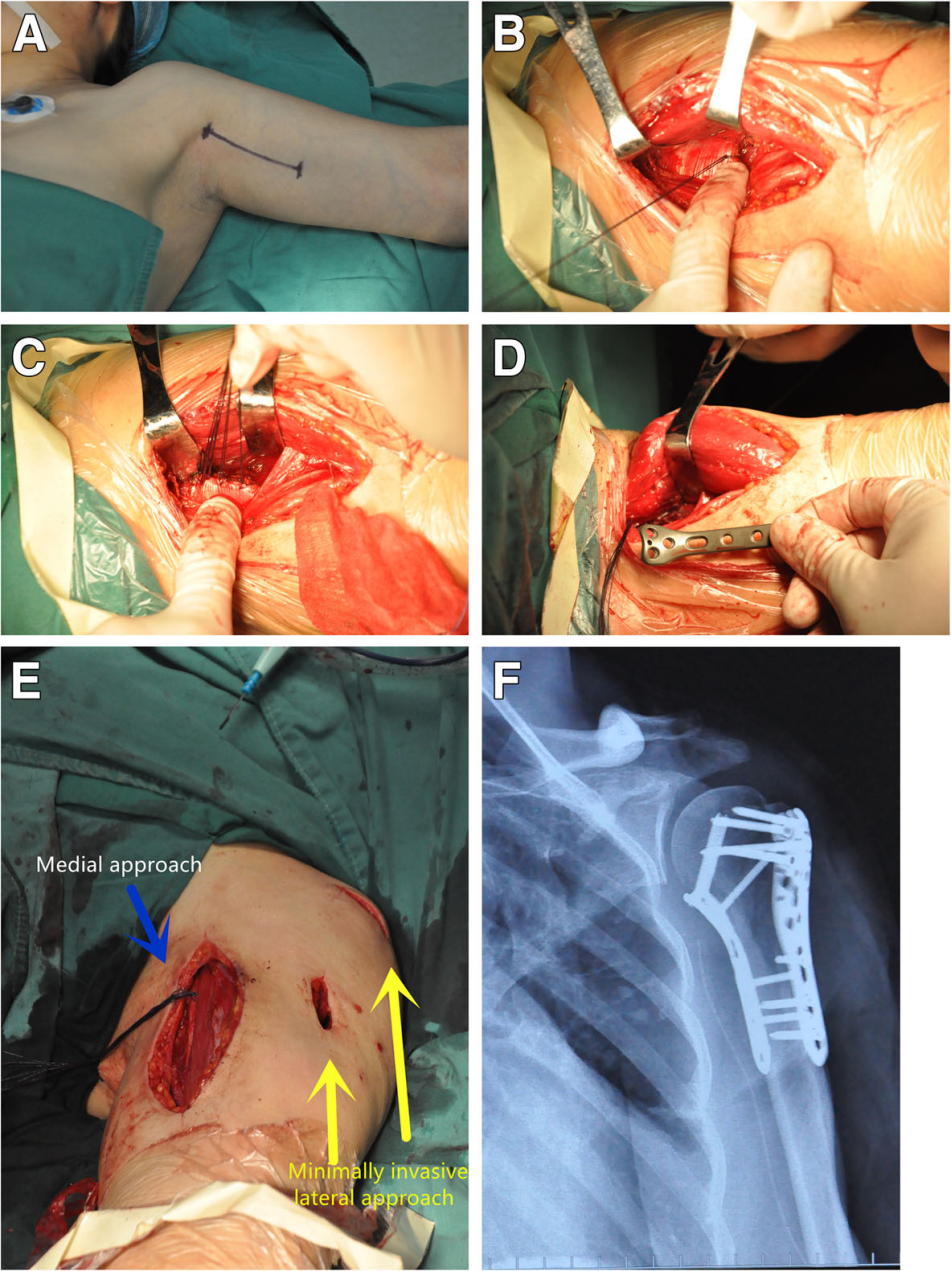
Intraoperative photograph of medial fixation of proximal humerus fractures. a The medial incision was marked with a pen before the operation. b The conjoined tendon of the latissimus dorsi and teres major muscles was identified. c The conjoined tendon was marked with suture traction and cut. d A K-wire was inserted through the incision for temporary fixation, and then a locking plate of appropriate length was inserted along the medial aspect of the humerus. e A PHILOS plate was used through a minimally invasive anterolateral approach. f Immediate postoperative radiographs
